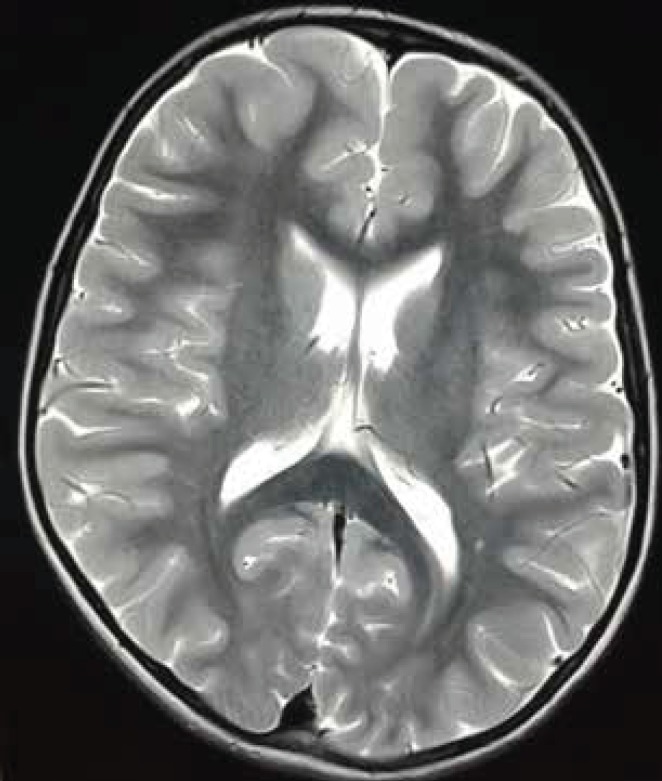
(post contrast) –A repeat MRI after 6months
